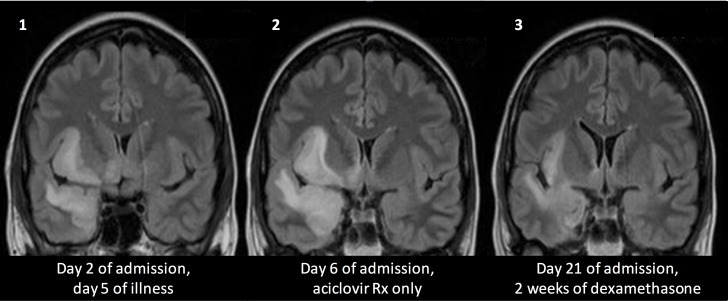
Coronal T2-weighted FLAIR images of a 45 year old woman with HSV encephalitis (Patient 3). On day 7 of admission a five-day course of corticosteroids was started. The same image slice is depicted from the day 2 scan (Image 1), from day 6 (Image 2) and from day 21 (Image 3)
